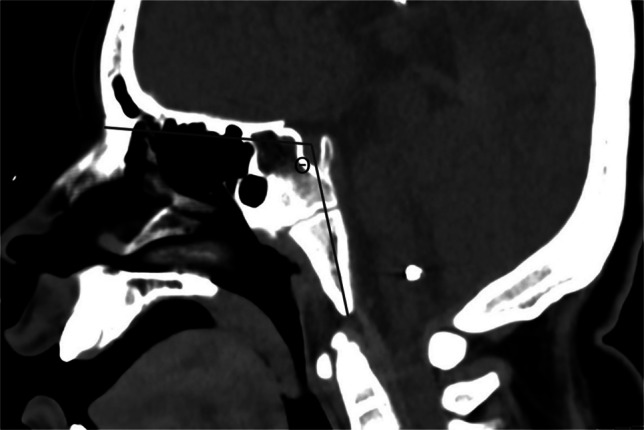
Sagittal CT of the brain in a 10-year-old male. Basilar kyphosis, also known as vertical dens, is when the basal angle is smaller than normative data (the converse of platybasia when the angle is larger than normative data). Basilar kyphosis is of no clinical significance
